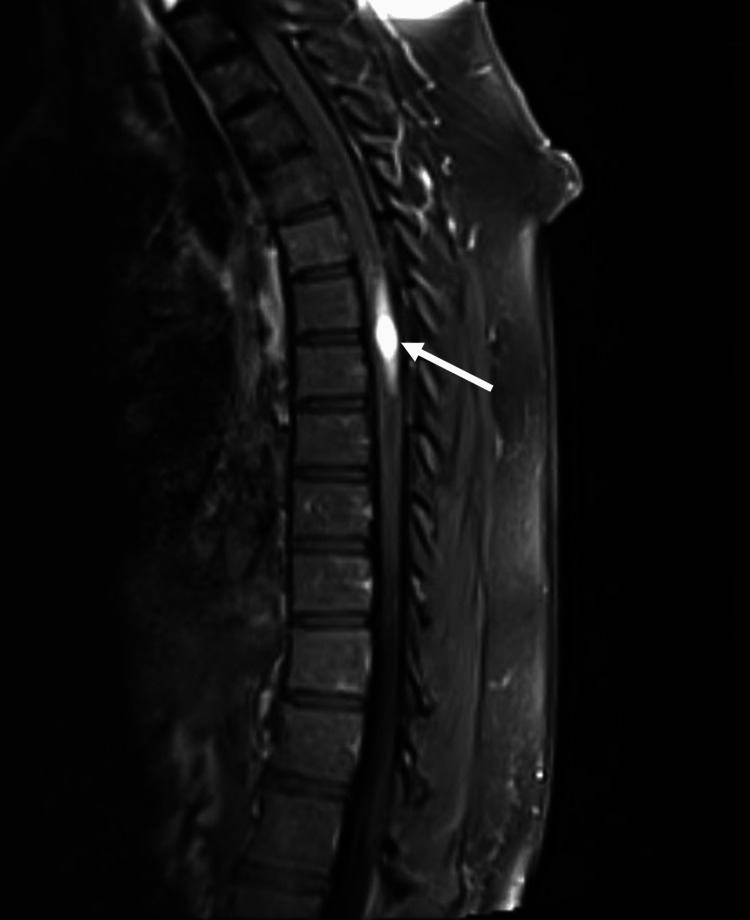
Sagittal MRI T1 sequence with gadolinium contrast demonstrating avidly enhancing intramedullary lesion at T6-7.
